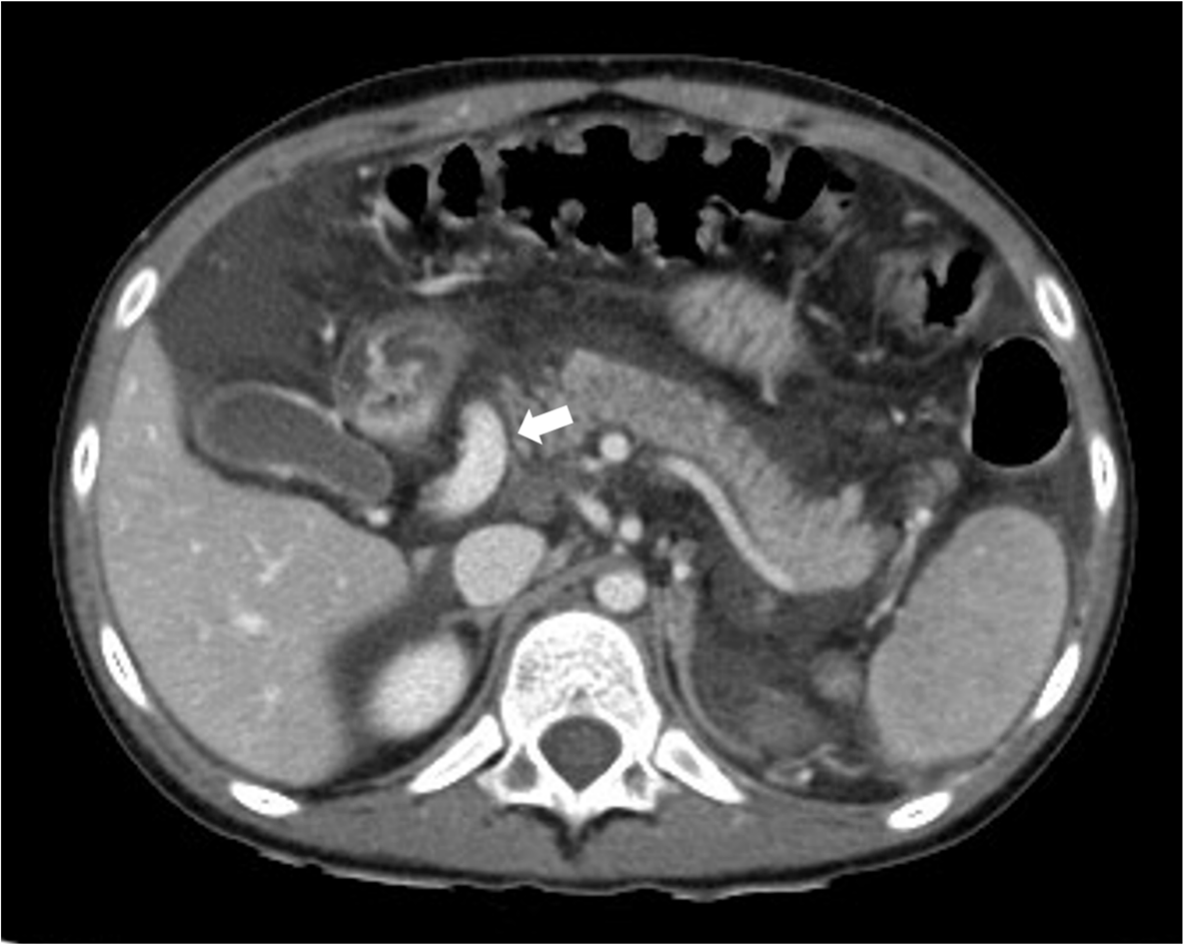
CTA of a 8-year old female who presentend with hematemesis due to variceal bleeding. Axial CT image in the portal venous phase shows an aberrant mesenteric retroduodenal venous collateral (arrow)
